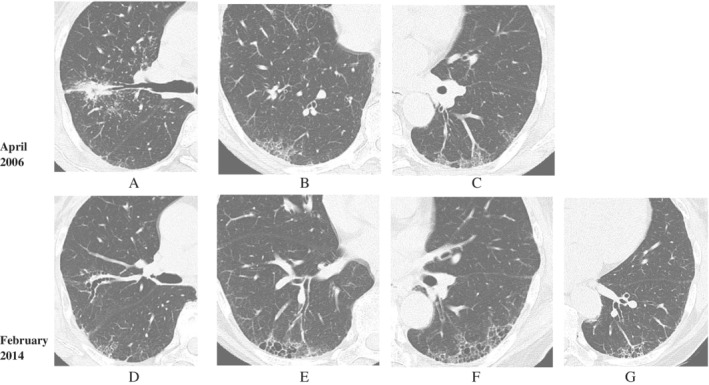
Computed tomography (CT) images taken in April 2006 (A–C). CT scans in April 2006 showed infiltrative shadows in both upper lobes and granular opacities distributed throughout the entire lung field (A), and ground‐glass or reticular pattern opacities in a wedge shape at bronchial bundle terminals on the posterior aspect of both lower lobes (B, C). CT images taken in February 2014 (D–G). CT scans in February 2014 showed the disappearance of infiltrative shadows and the appearance of bronchial dilation in both upper lobes (D). Ground‐glass opacities disappeared, and honeycomb‐like structures continuous with dilated bronchioles of which walls were thickened and appeared in both lower lobes (E–G).
